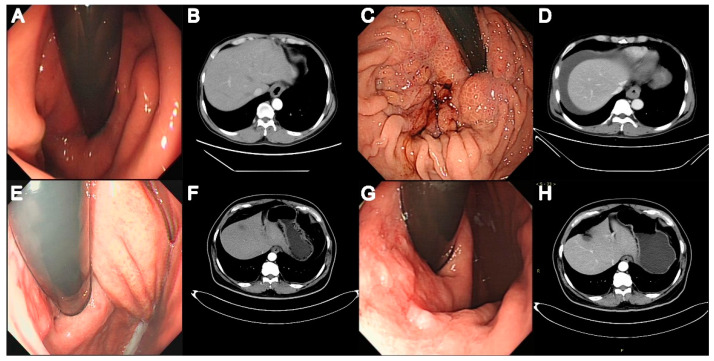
Endoscopic and computed tomography (CT) scan images of two patients with EGJOO and subsequent adenocarcinoma of the esophagogastric junction (AEG). Patient 1 was diagnosed with cardia inflammation using endoscopy (A) and pathology. Thickened cardiac wall and slight stricture of the esophagus are seen on CT (B) performed at the initial visit. Six months later, AEG (T3N0M1) was diagnosed. Endoscopy shows coarse, hyperemic, and easily bleeding cardia mucosa (C). CT shows an obviously thickened cardiac wall (D). In Patient 2, endoscopy performed at the initial visit (E) shows cardia mucosa hyperemia; CT (F) shows a slightly thickened cardiac wall. Endoscopy (G) performed one-and-a-half years later shows high-grade localized intraepithelial neoplasia in the gastric mucosa; this was confirmed on pathology. CT shows thickened and enhanced cardiac wall (H).
